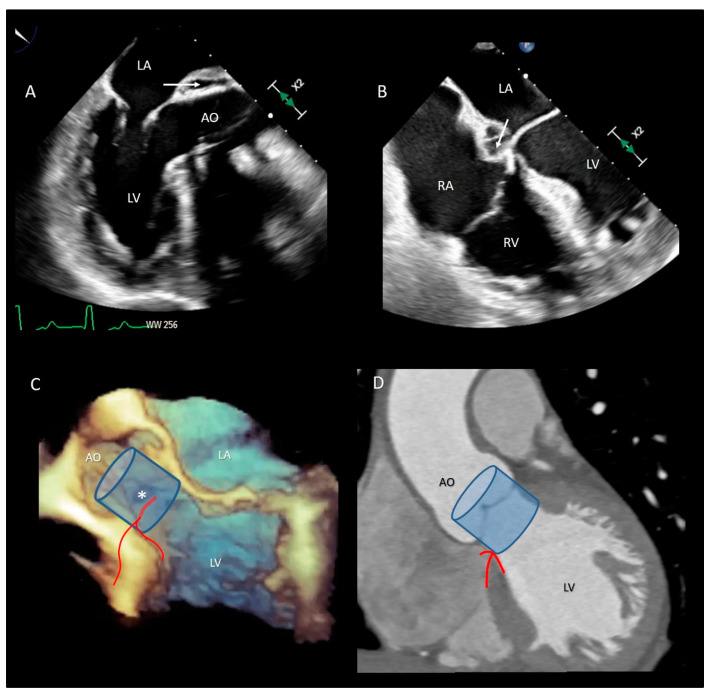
(A) The 2D TEE in long axis-view showing a peri-annular aortic abscess (arrow). (B) Same case of panel A showing the abscess may extend posteriorly on the crux cordis (arrow). (C) The 3D TEE in long-axis view. The red curve lines mark the position of His bundle immediately under the MS (asterisk) and its bifurcation. (D) CT multiplanar reconstruction showing the course of the His bundle and its branches (red lines). The light blue cylinders are representative of the position of the implanted valve. AO = aorta; LV = left ventricle; LA = left atrium; RA = right atrium; RV = right ventricle.
